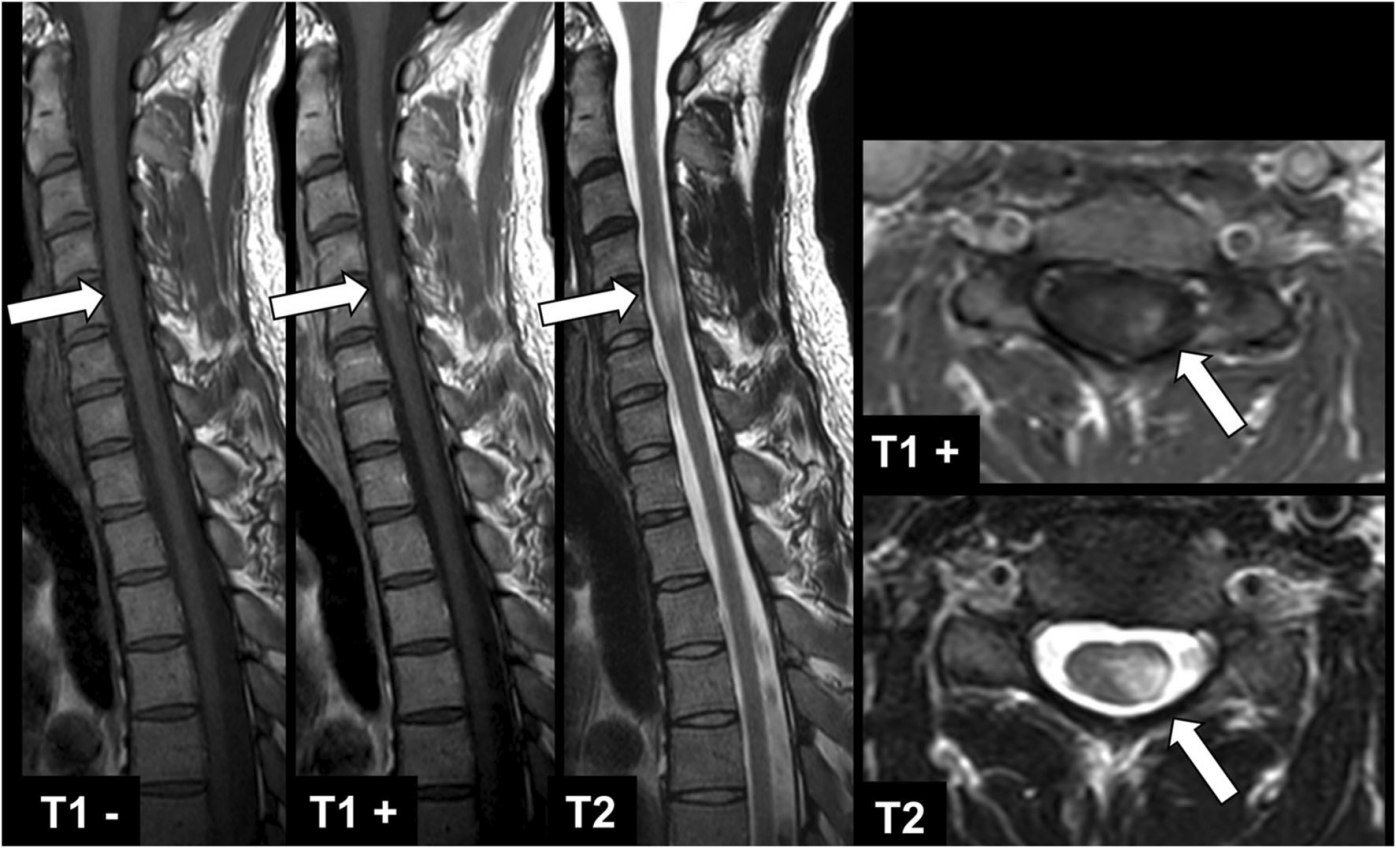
MRI of a 34-year-old male patient with MS with sagittal pre- and post-contrast T1-w, sagittal T2-w, axial post-contrast T1-w, and axial T2-w images. Note the typical contrast-enhancing lesion in the cervical spinal cord at level C5 with isointense signal in pre-contrast T1-w images, hyperintense signal in T2-w images, and strong contrast enhancement (arrows)
